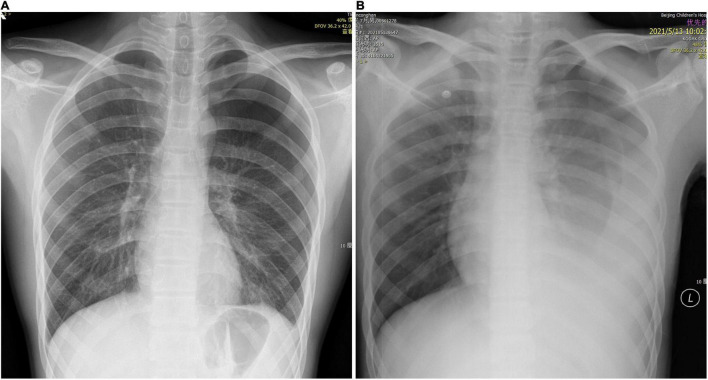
Chest X-ray. (A) Left pneumothorax volume of approximately 30%. (B) Left pneumothorax with massive pleural effusion.
